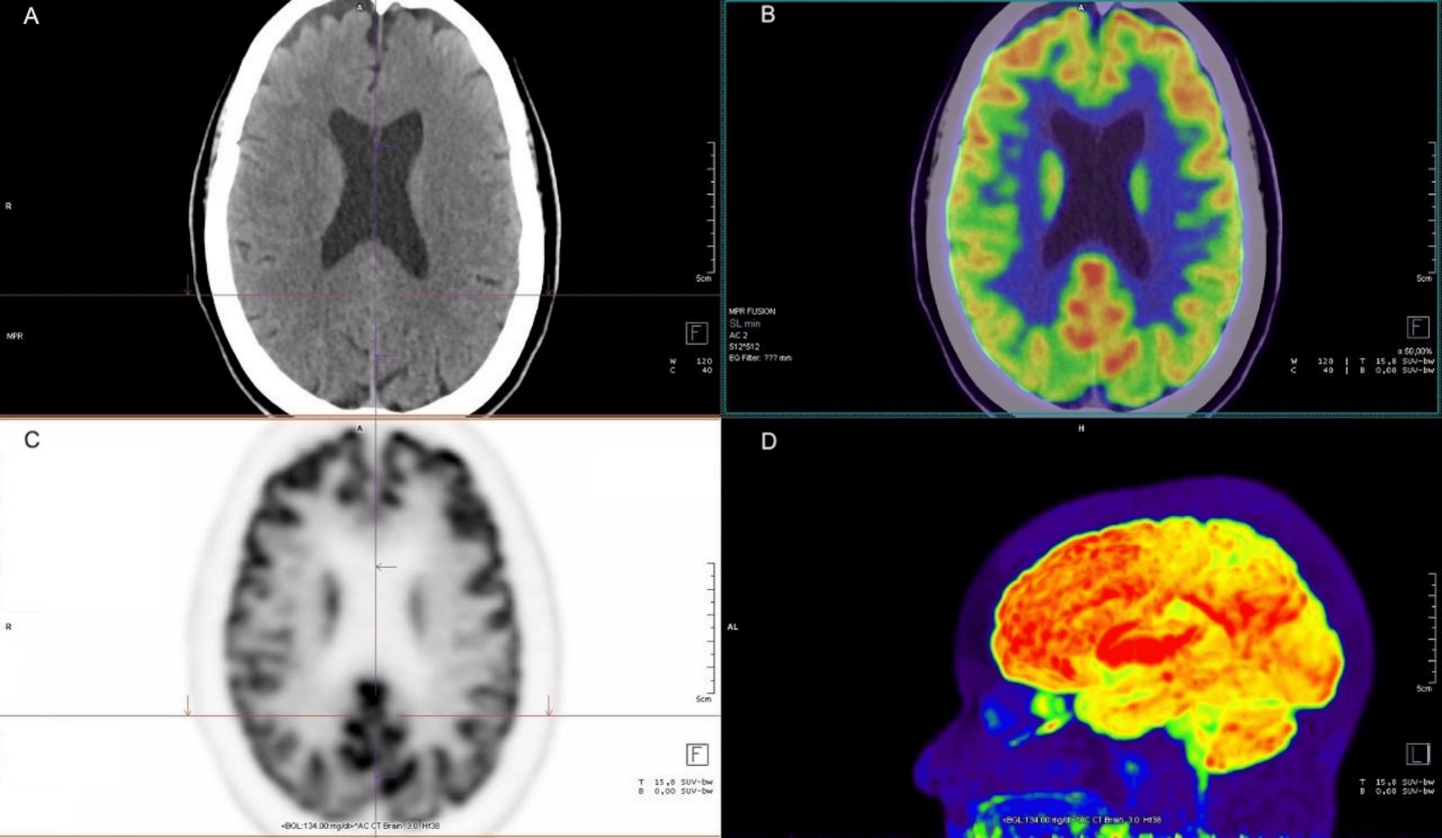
(A) Axial cranial CT scan showing no significant structural abnormalities. (B) Axial ¹⁸F-fluorodeoxyglucose (¹⁸F-FDG) brain PET image showing the cingulate island sign, with crosshairs marking the area of preserved cingulate metabolism. (C) Fused ¹⁸F-FDG PET/CT brain images. (D) Lateral view maximum intensity projection (MIP) image of the ¹⁸F-FDG brain PET study.
